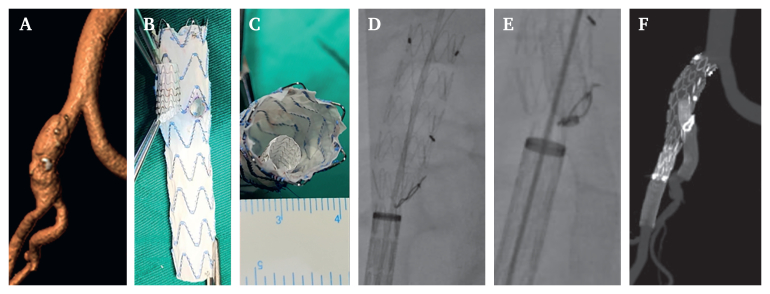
Physician modified inner branch iliac branch device (PM-IIBD) implantation process: (A) pre-operative computed tomography angiography showing the narrow inner lumen common iliac artery aneurysm; (B, C) PM-IIBD configuration; (D) partial release of the PM-IIBD with the inner branch opening aligned to the internal iliac artery; € reconstruction of the internal iliac artery via the inner branch of the inner branch iliac branch device; (H) post-operative computed tomography angiography at 12 month follow up.
